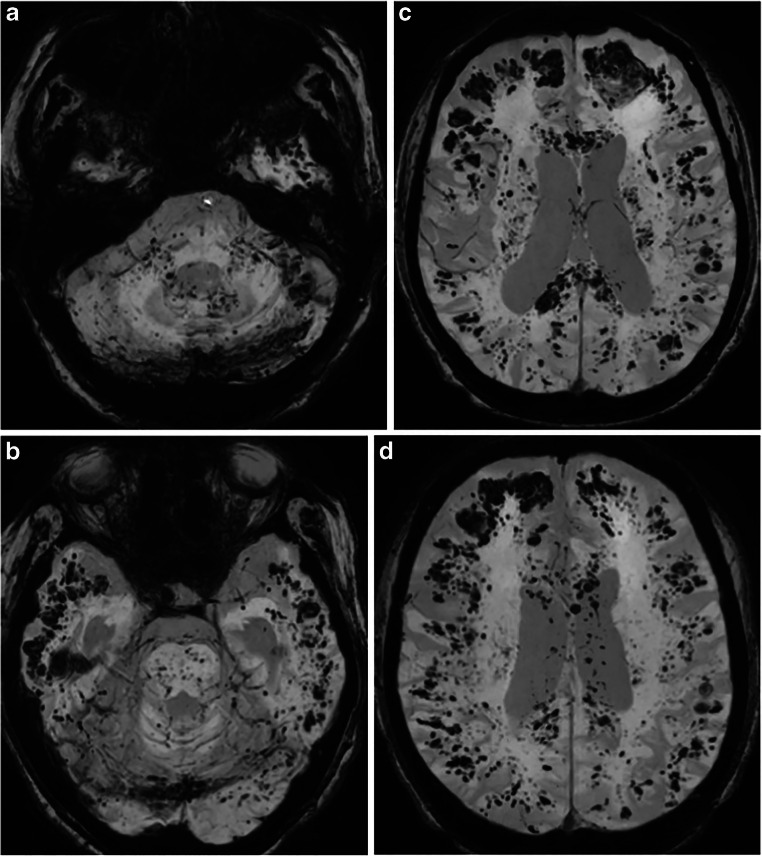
Susceptibility-weighted MR images (MRI) of the brain in a 55-year-old female patient with ARDS after abdominal surgery, demonstrate diffuse susceptibilities (type I) in the cerebellum (a), pons (b), gray and white matter interface (b,c,d), corpus callosum (b)
